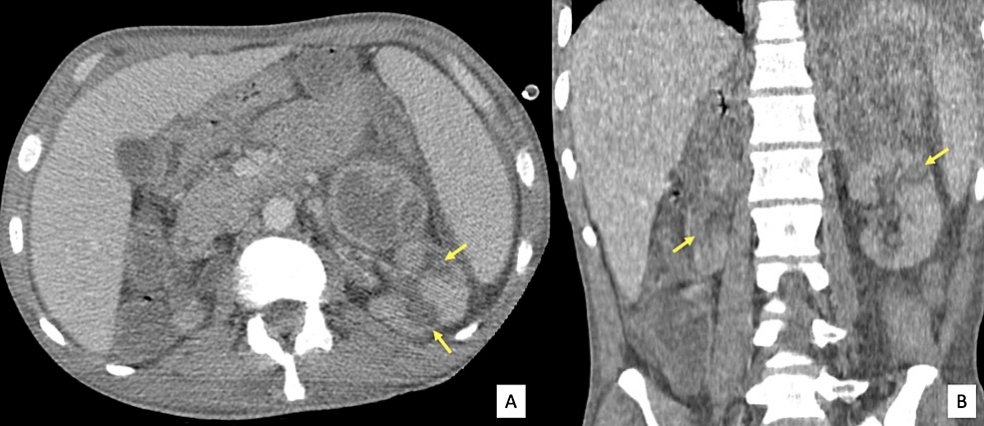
Axial (A) and coronal (B) CT images Wedge-shaped hypodense lesions in both kidneys (arrows) along with perinephric fat stranding.
